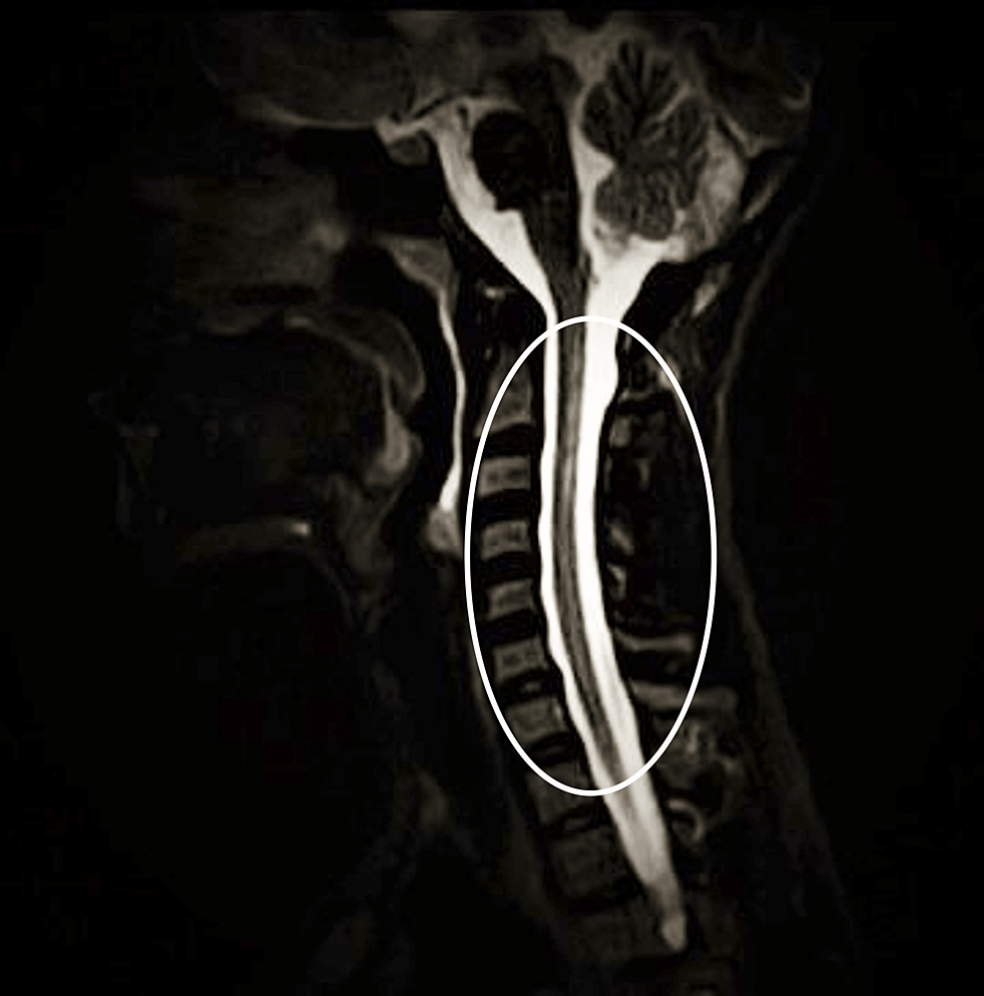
A sagittal T2-weighted MR image demonstrates hyperintensity of the cervical cord
